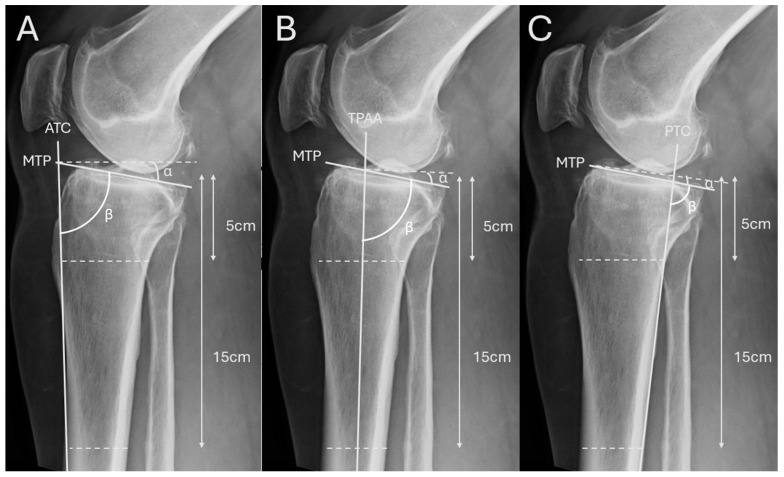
Radiograph-based measurement methods of the PTS. (A) Anterior Tibial Cortex (ATC) method: The anterior tibial cortex serves as the reference axis. A line is drawn along the anterior cortical border of the tibia, and the medial tibial plateau (MTP) line is placed tangentially to the joint surface. The PTS is defined by the angle (α) between the MTP and a line perpendicular to the ATC. Angle β is measured between MTP and the reference axis ATC. Reference points are located 5 cm and 15 cm distal to the tibial plateau. (B) Tibial Proximal Anatomical Axis (TPAA) method: The proximal tibial anatomical axis (TPAA) represents the anatomical shaft axis of the proximal tibia. To define it, transverse lines are drawn between the anterior and posterior cortices at two levels (5 cm and 15 cm distal to the plateau), and their midpoints are connected to form the TPAA. The angle (α) between the MTP and a line perpendicular to the TPAA corresponds to the PTS. Angle β is measured between MTP and the reference axis TPAA. (C) Posterior Tibial Cortex (PTC) method: The posterior tibial cortex is used as the reference axis. A line is drawn along the posterior tibial border, and the PTS is measured as the angle (α) between the MTP and a line perpendicular to the PTC. Angle β is measured between MTP and the reference axis PTC. As in the previous methods, reference distances of 5 cm and 15 cm distal to the tibial plateau are used for axis definition.
